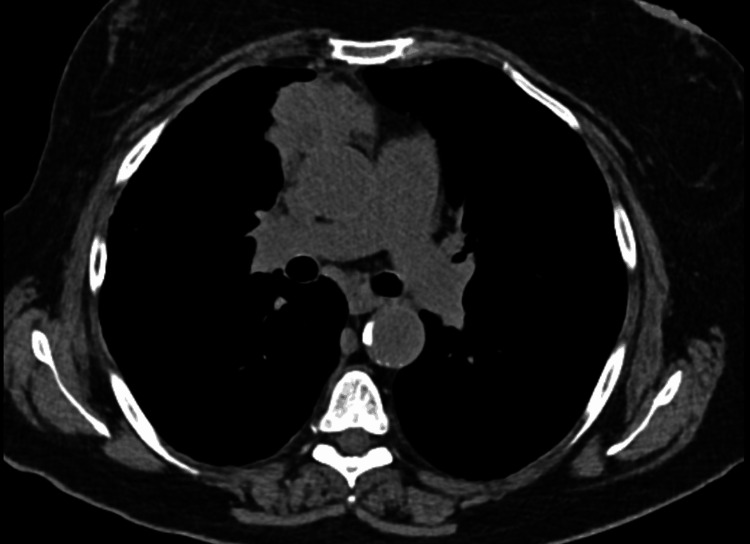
CT chest showing a large increase in the size of anterior mediastinal mass
